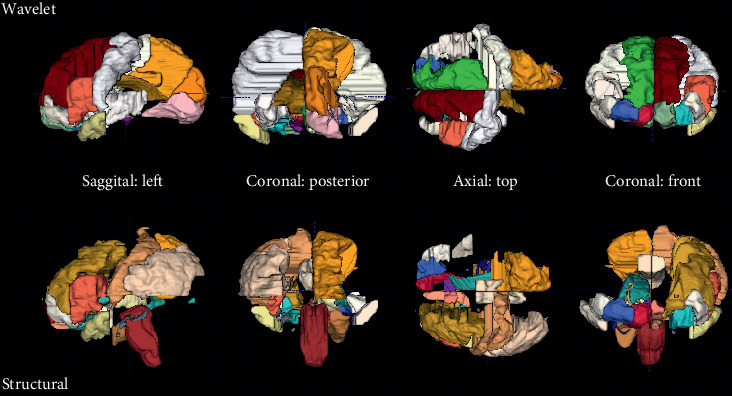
MRI regions selected for prediction of divided attention decline. For the prediction of divided attention decline, wavelet MRI features were selected from the following regions: insula left, corpus callosum, third ventricle, precentral gyrus left, straight gyrus right, anterior orbital gyrus right, bilateral inferior frontal gyrus, bilateral superior frontal gyrus, postcentral gyrus right, superior parietal gyrus right, lingual gyrus right, cuneus right, bilateral medial orbital gyrus, bilateral lateral orbital gyrus, posterior orbital gyrus left, bilateral substantia nigra, bilateral subgenual frontal cortex, bilateral subcallosal area, bilateral pre-subgenual frontal cortex, and bilateral superior temporal gyrus anterior part. For the prediction of divided attention decline by structural MRI volumetry, the following regions were selected: parahippocampal and ambient gyrus right, brainstem excluding substantia nigra, bilateral posterior cingulate gyrus, middle frontal gyrus left, inferolateral remainder parietal lobe left, nucleus accumbens right, thalamus right, lateral ventricle including temporal horn right, lateral ventricle temporal horn left, straight gyrus left, anterior orbital gyrus right, inferior frontal gyrus left, postcentral gyrus left, superior parietal gyrus right, medial orbital gyrus right, bilateral lateral orbital gyrus, posterior orbital gyrus left, bilateral subgenual frontal cortex, subcallosal area right, and bilateral superior temporal gyrus anterior part.
